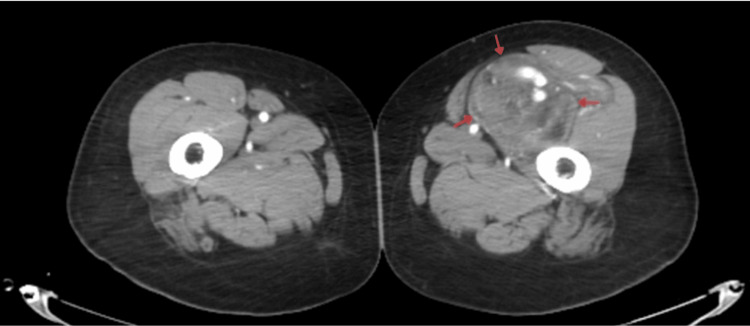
CT angiogram of the thighs (axial view) A large, enhancing intermuscular mass is present in the anteromedial compartment of the left thigh. Multiple dilated feeding vessels and prominent intralesional vascular channels are seen, arising from branches of the profunda femoris artery.
